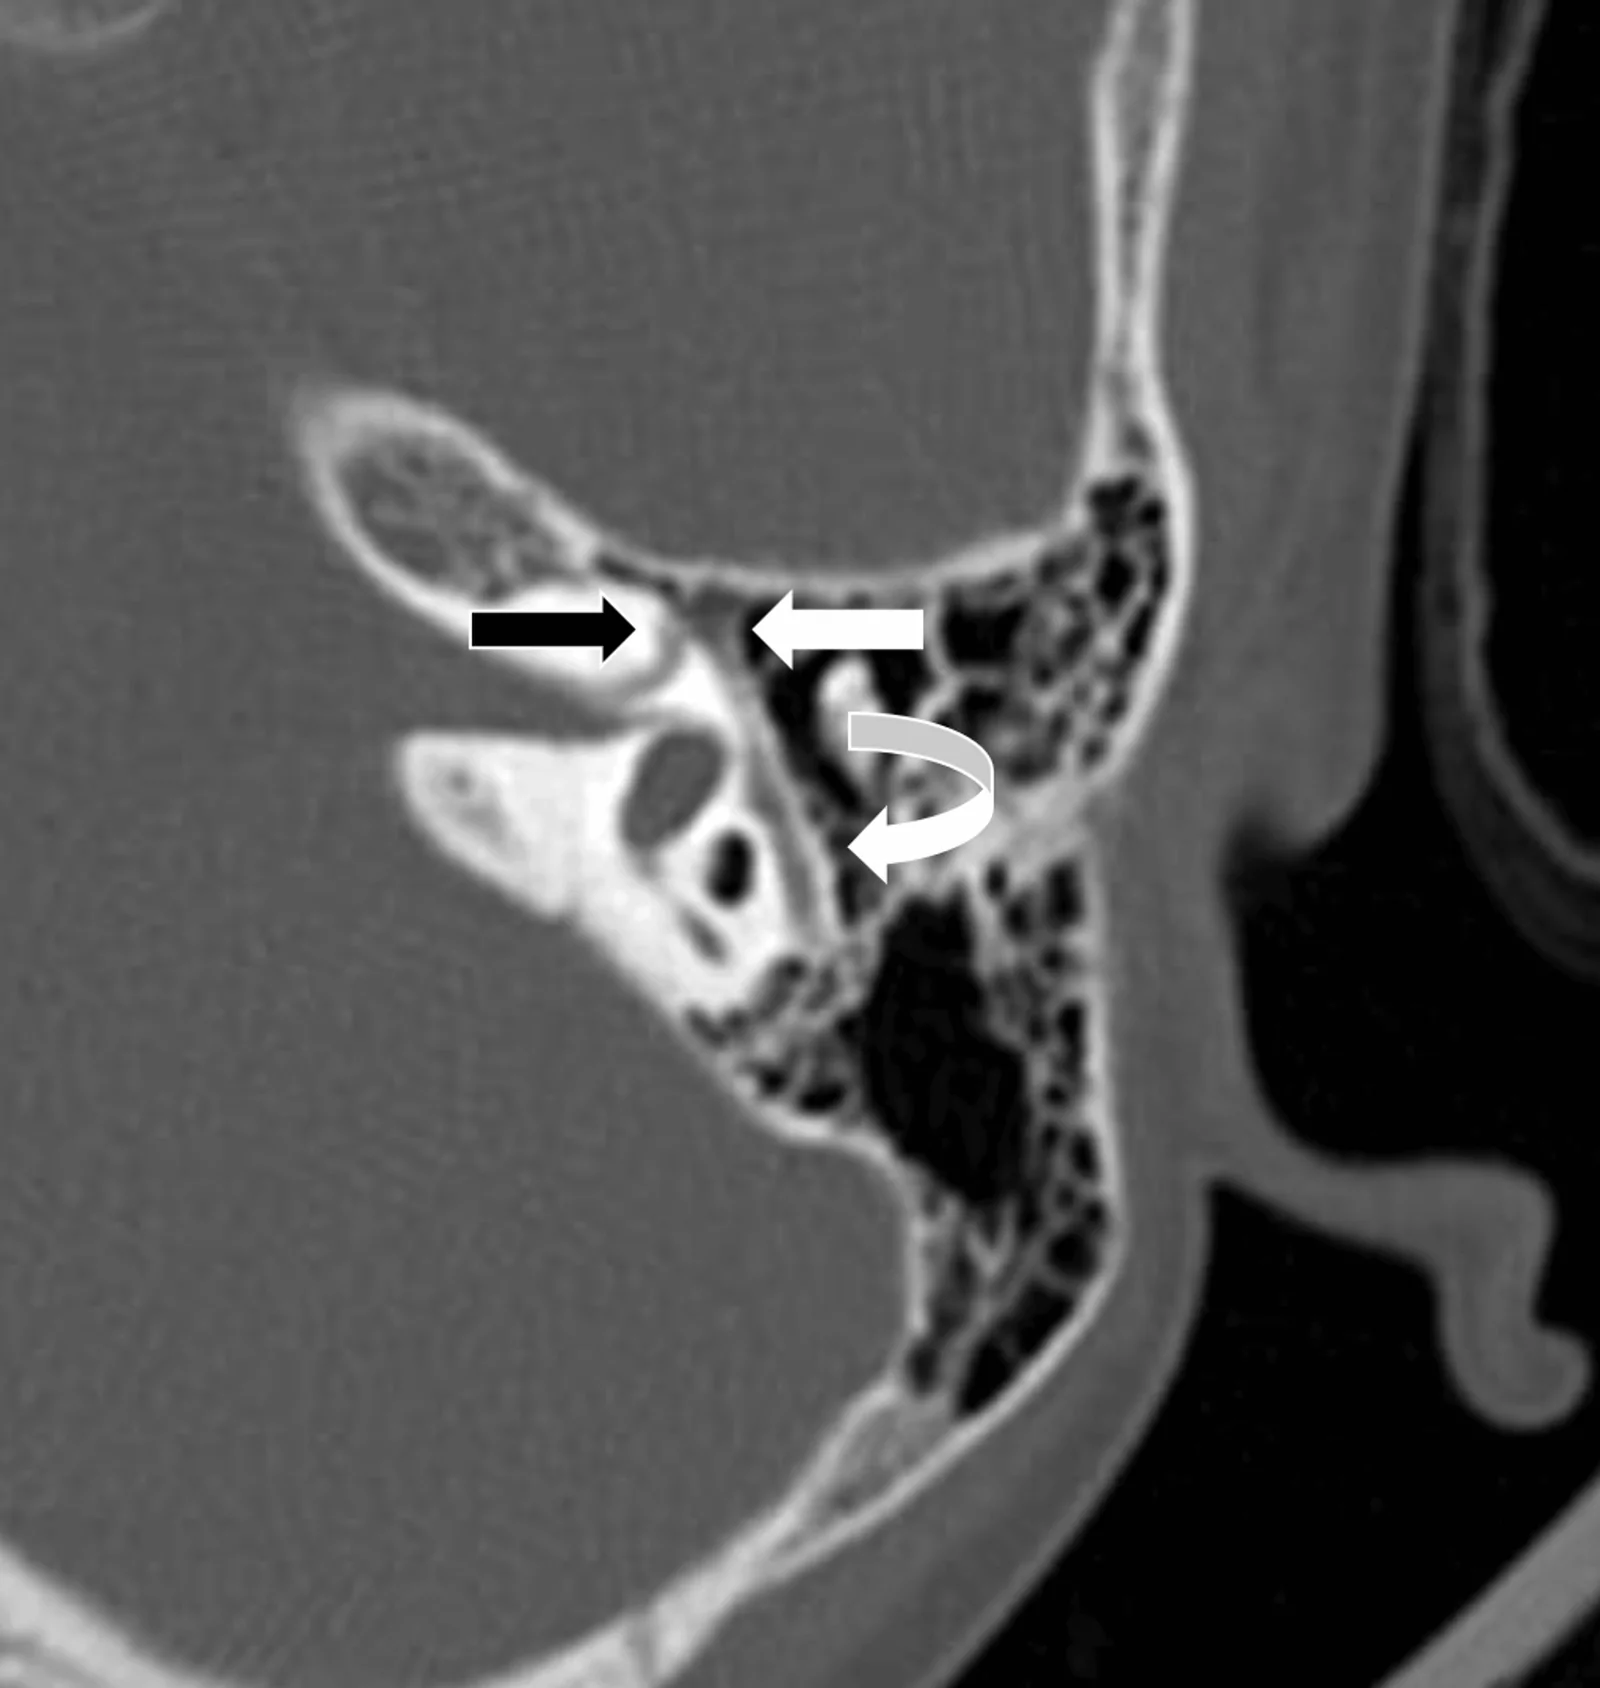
High-resolution computed tomography (HRCT) of the left temporal bone - axial section The images demonstrate a normal, well-defined bony facial canal for comparison. Black straight arrow: labyrinthine part of the facial nerve canal; white straight arrow: first genu of the facial nerve canal; curved arrow: tympanic part of the facial nerve canal
